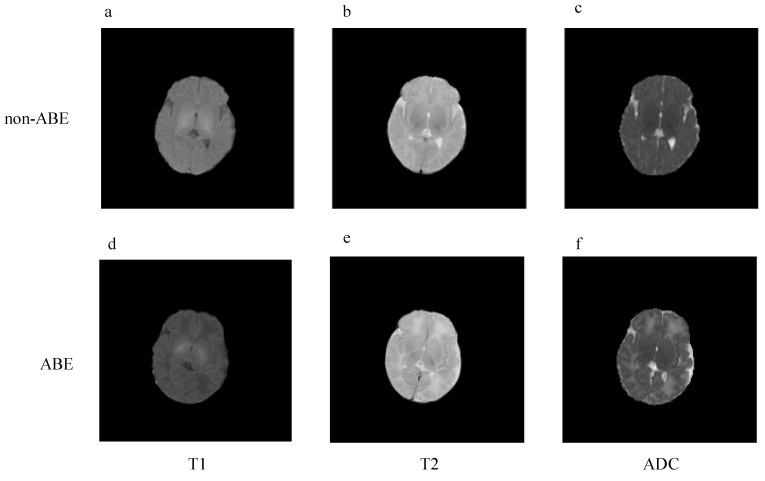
Axial images of three modalities of MRI: (a–c) represent T1, T2, and ADC images of non-ABE neonates, respectively; (d–f) represent T1, T2, and ADC images of ABE neonates, respectively.
